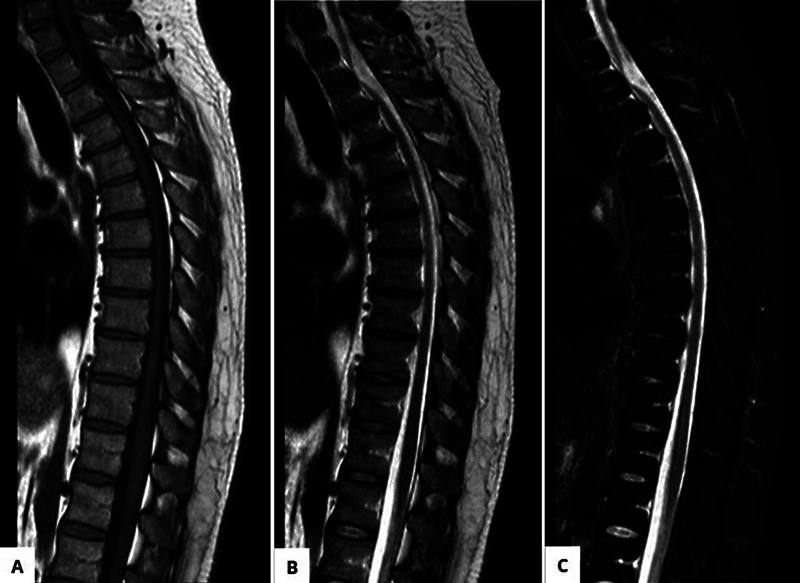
Magnetic resonance imaging (MRI) of the spine. (
